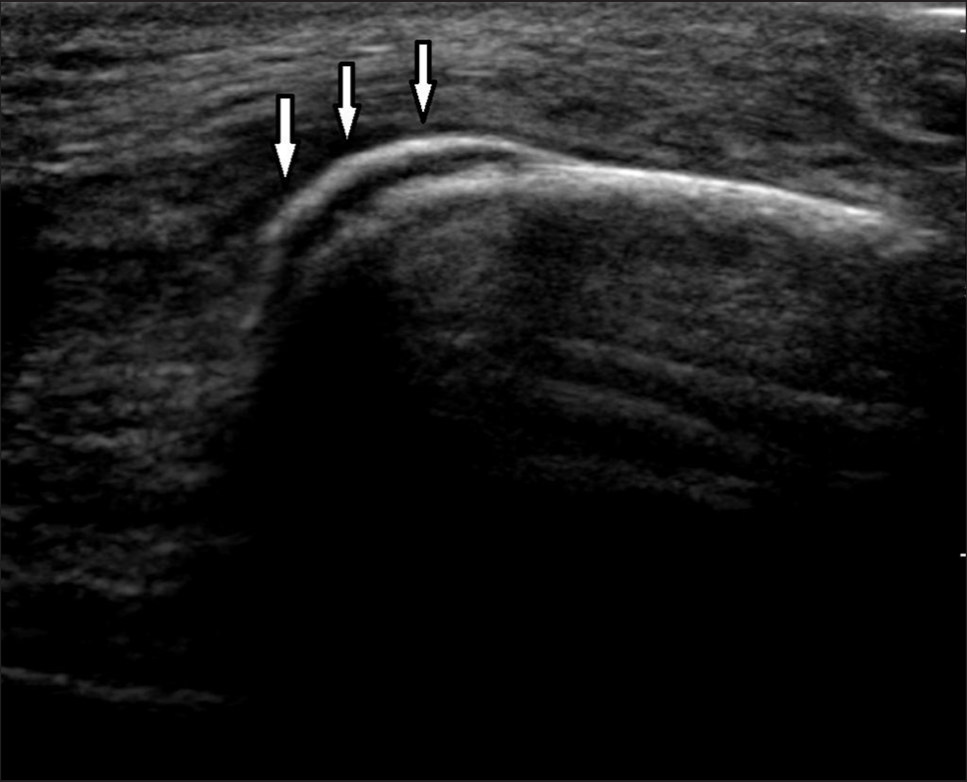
Ultrasound of the suprapatellar region shows a crescent-shaped echogenic fragment at the upper pole of the patella.
